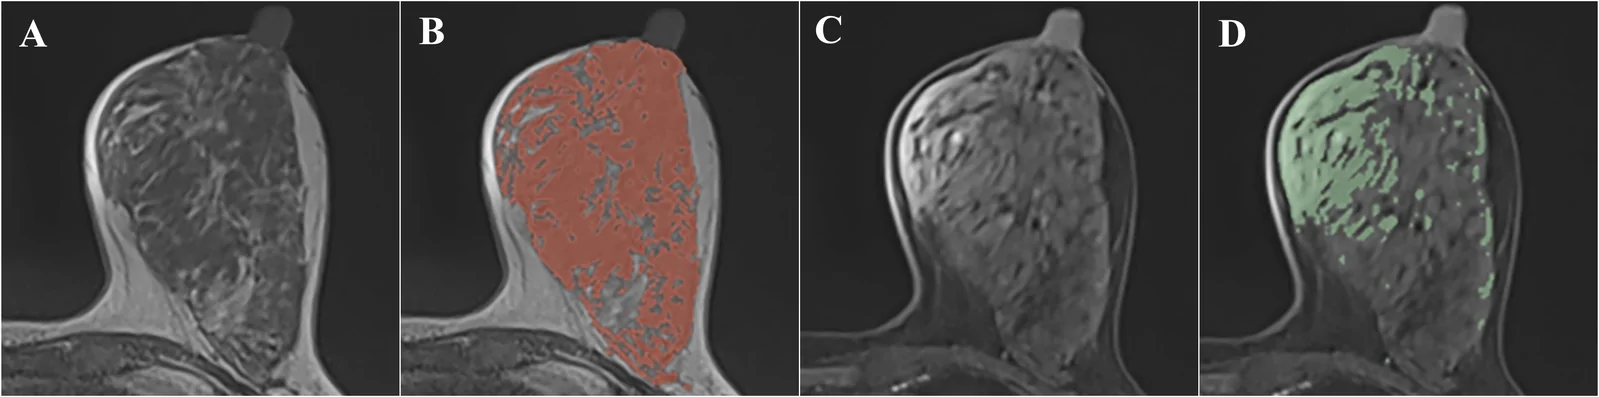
The proportion of background parenchymal enhancement of the patient was 42.9%. (A) Axial T1-weighted precontrast image with the contralateral breast selected as the region of interest. (B) Segmentation of fibroglandular tissue (highlighted in red) on the T1-weighted image. (C) Early postcontrast phase (second dynamic phase) image of the contralateral breast. (D) Segmentation of enhancing BPE (highlighted in green) on the early postcontrast image.
